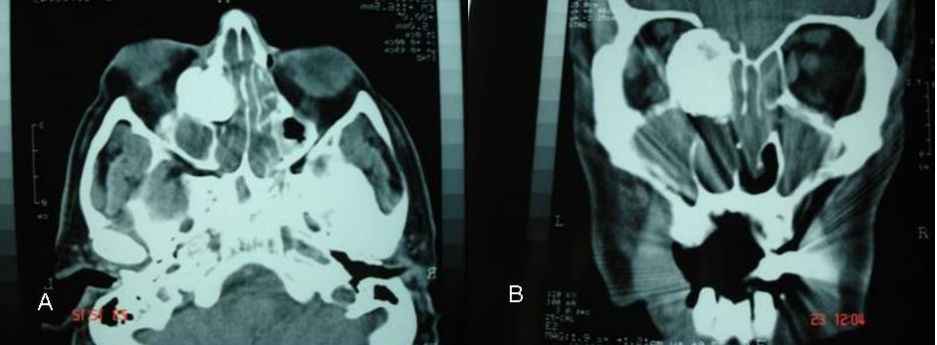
Preoperative computed tomography a) axial b) coronal.
